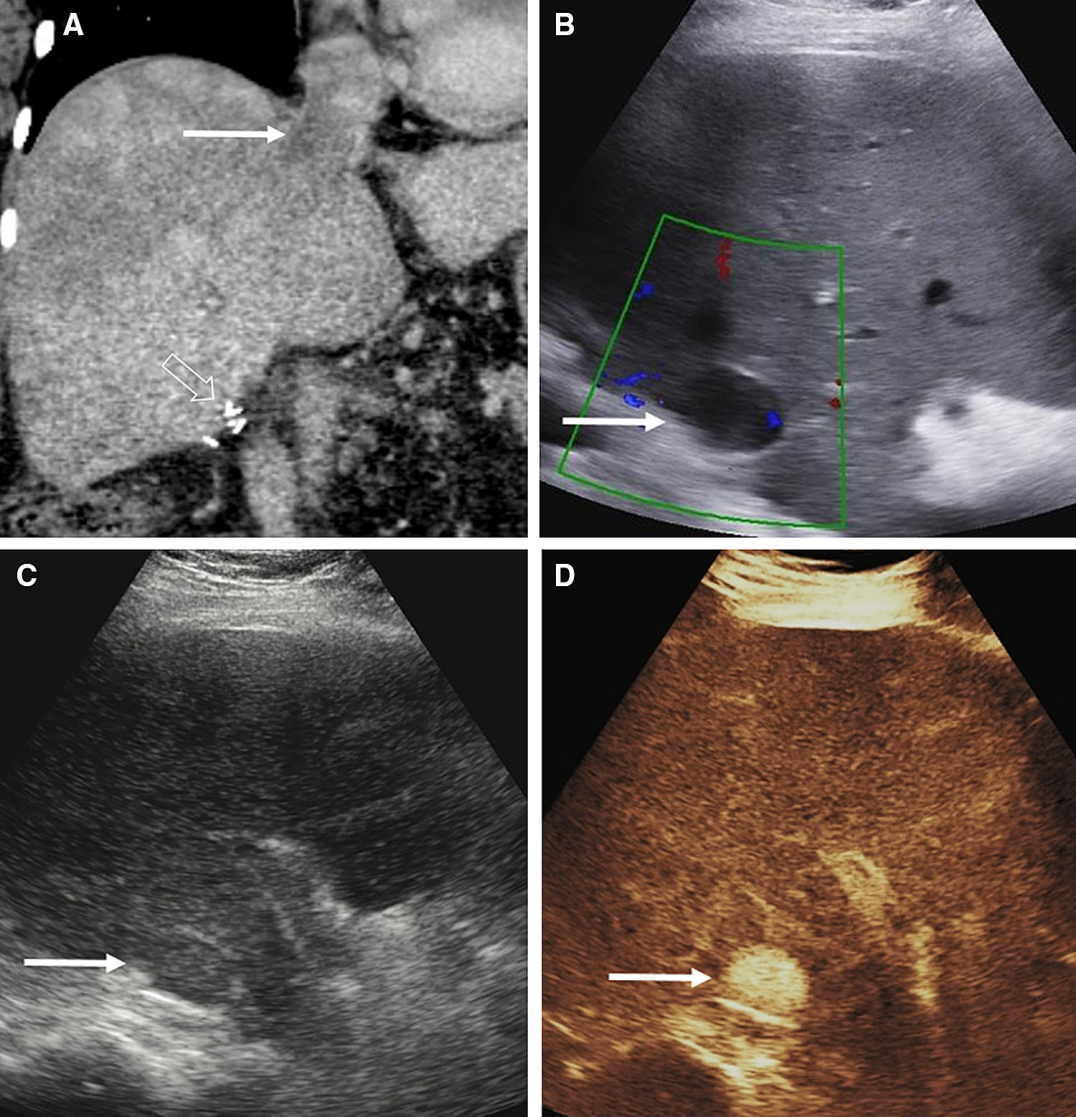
Patient with history of invasive retroperitoneal sarcoma compressing on IVC which was resected (surgical clips, open arrow). She subsequently presented with bilateral lower limb pitting edema. Imaging studies were performed to exclude IVC thrombus (A) coronal CECT (B) Color Doppler US and (C) Gray-scale US demonstrate near occlusive thrombus within the IVC (arrows). (D) The thrombus shows enhancement with microbubble ultrasound contrast imaging in keeping with tumor thrombus (arrow)
